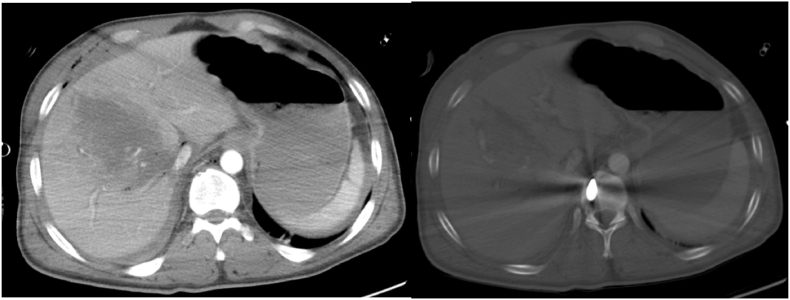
Axial images from a post contrast CT scan of the upper abdomen. Left – soft tissue window. Right – bone window. Fragment penetration through the right side of the abdomen, disrupting the liver. The right lobe shows disruption with haematoma and small locules of gas. Further small locules of gas are seen anterior to the liver in the right upper quadrant within the abdomen and also within the subcutaneous soft tissues of the right upper abdominal wall. Fragment lies in the right of the vertebral body having passed through the liver. Note this is a post contrast CT scan – in the post-mortem setting non-contrasted CT scanning is most commonly performed. This does not represent an actual casualty from the Arena bombing.
